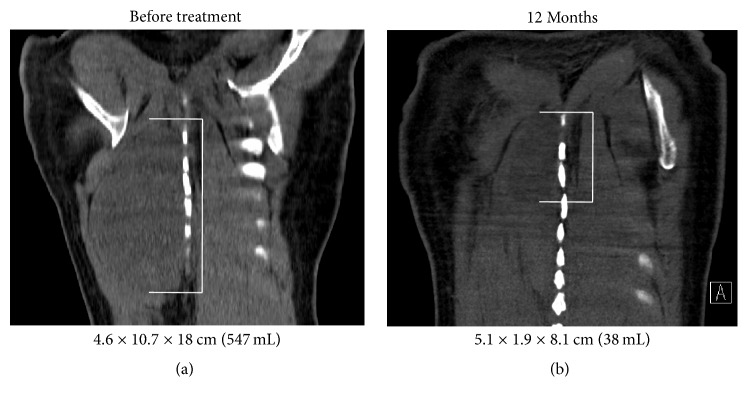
Imaging of Posterior Thorax Desmoid Treatment Response. Coronal CT of a right paraspinal desmoid tumor before (a) and 12 months after (b) completing radiation therapy to 50 Gy in 25 fractions with concurrent imatinib demonstrating a significant reduction in tumor volume. The tumor is delineated with white brackets.
